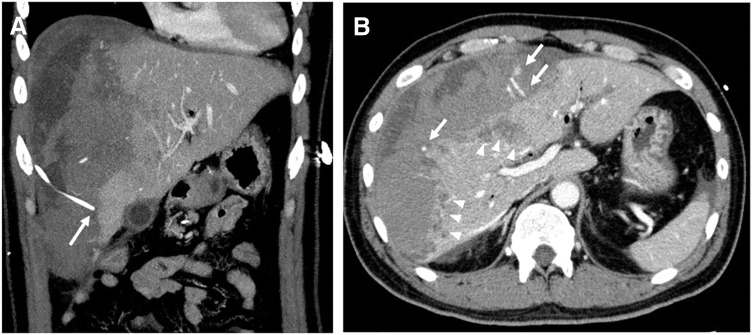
Contrast-enhanced CT of the abdomen. (A) The PTGBD catheter tip (arrow) is displaced into the subcapsular space of the right hepatic lobe, with a large subcapsular hematoma. (B) The hematoma causes marked mass effect on the hepatic parenchyma with collapse of the inferior vena cava and multiple adjacent non-enhancing hepatic areas (arrowheads) suggestive of ischemia. Several foci of active contrast extravasation (arrows) are also evident along the hepatic surface. PTGBD, percutaneous transhepatic gallbladder drainage
